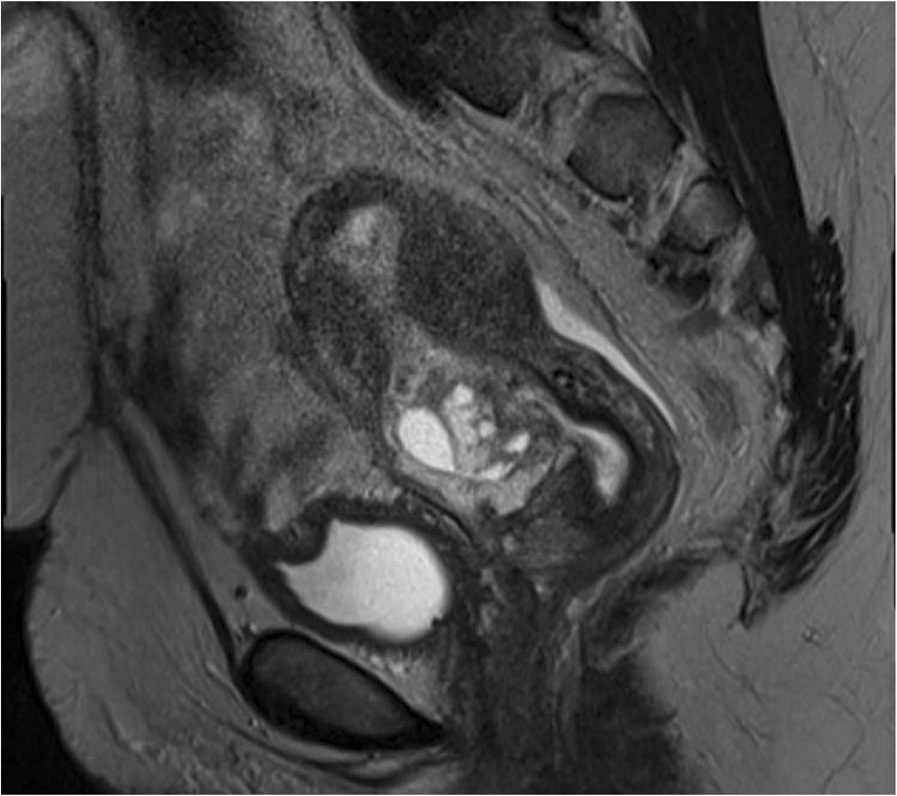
Magnetic resonance imaging of the pelvis, sagittal plane. Ectopic gestation can be seen within the anterior myometrial defect
